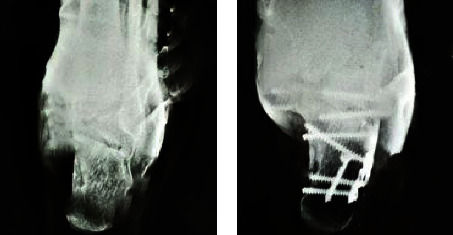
MILA surgery: (a) preoperative left calcaneus axial X-ray slice; (b) postoperative left calcaneus axial X-ray slice.
